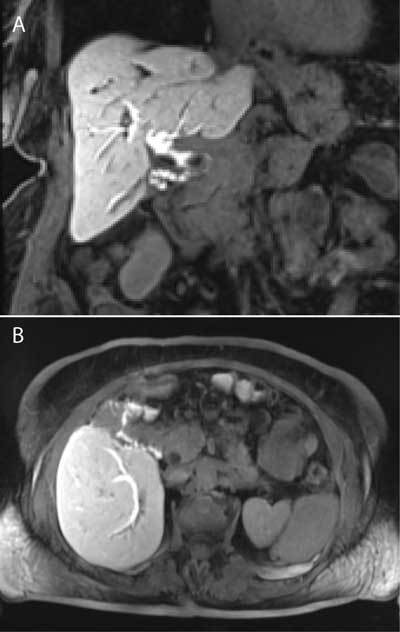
Coronal Fat-suppressed T1-weighted image (A) 90 mm after administration of Primovist® shows the filling of the intrahepatic bile ducts, common hepatic duct and cystic duct (black arrow). Axial Maximum Intensity Projection reconstruction (B) reveals the fistulous tract (white arrow) and presence of contrast in the transverse colon (black arrow).
